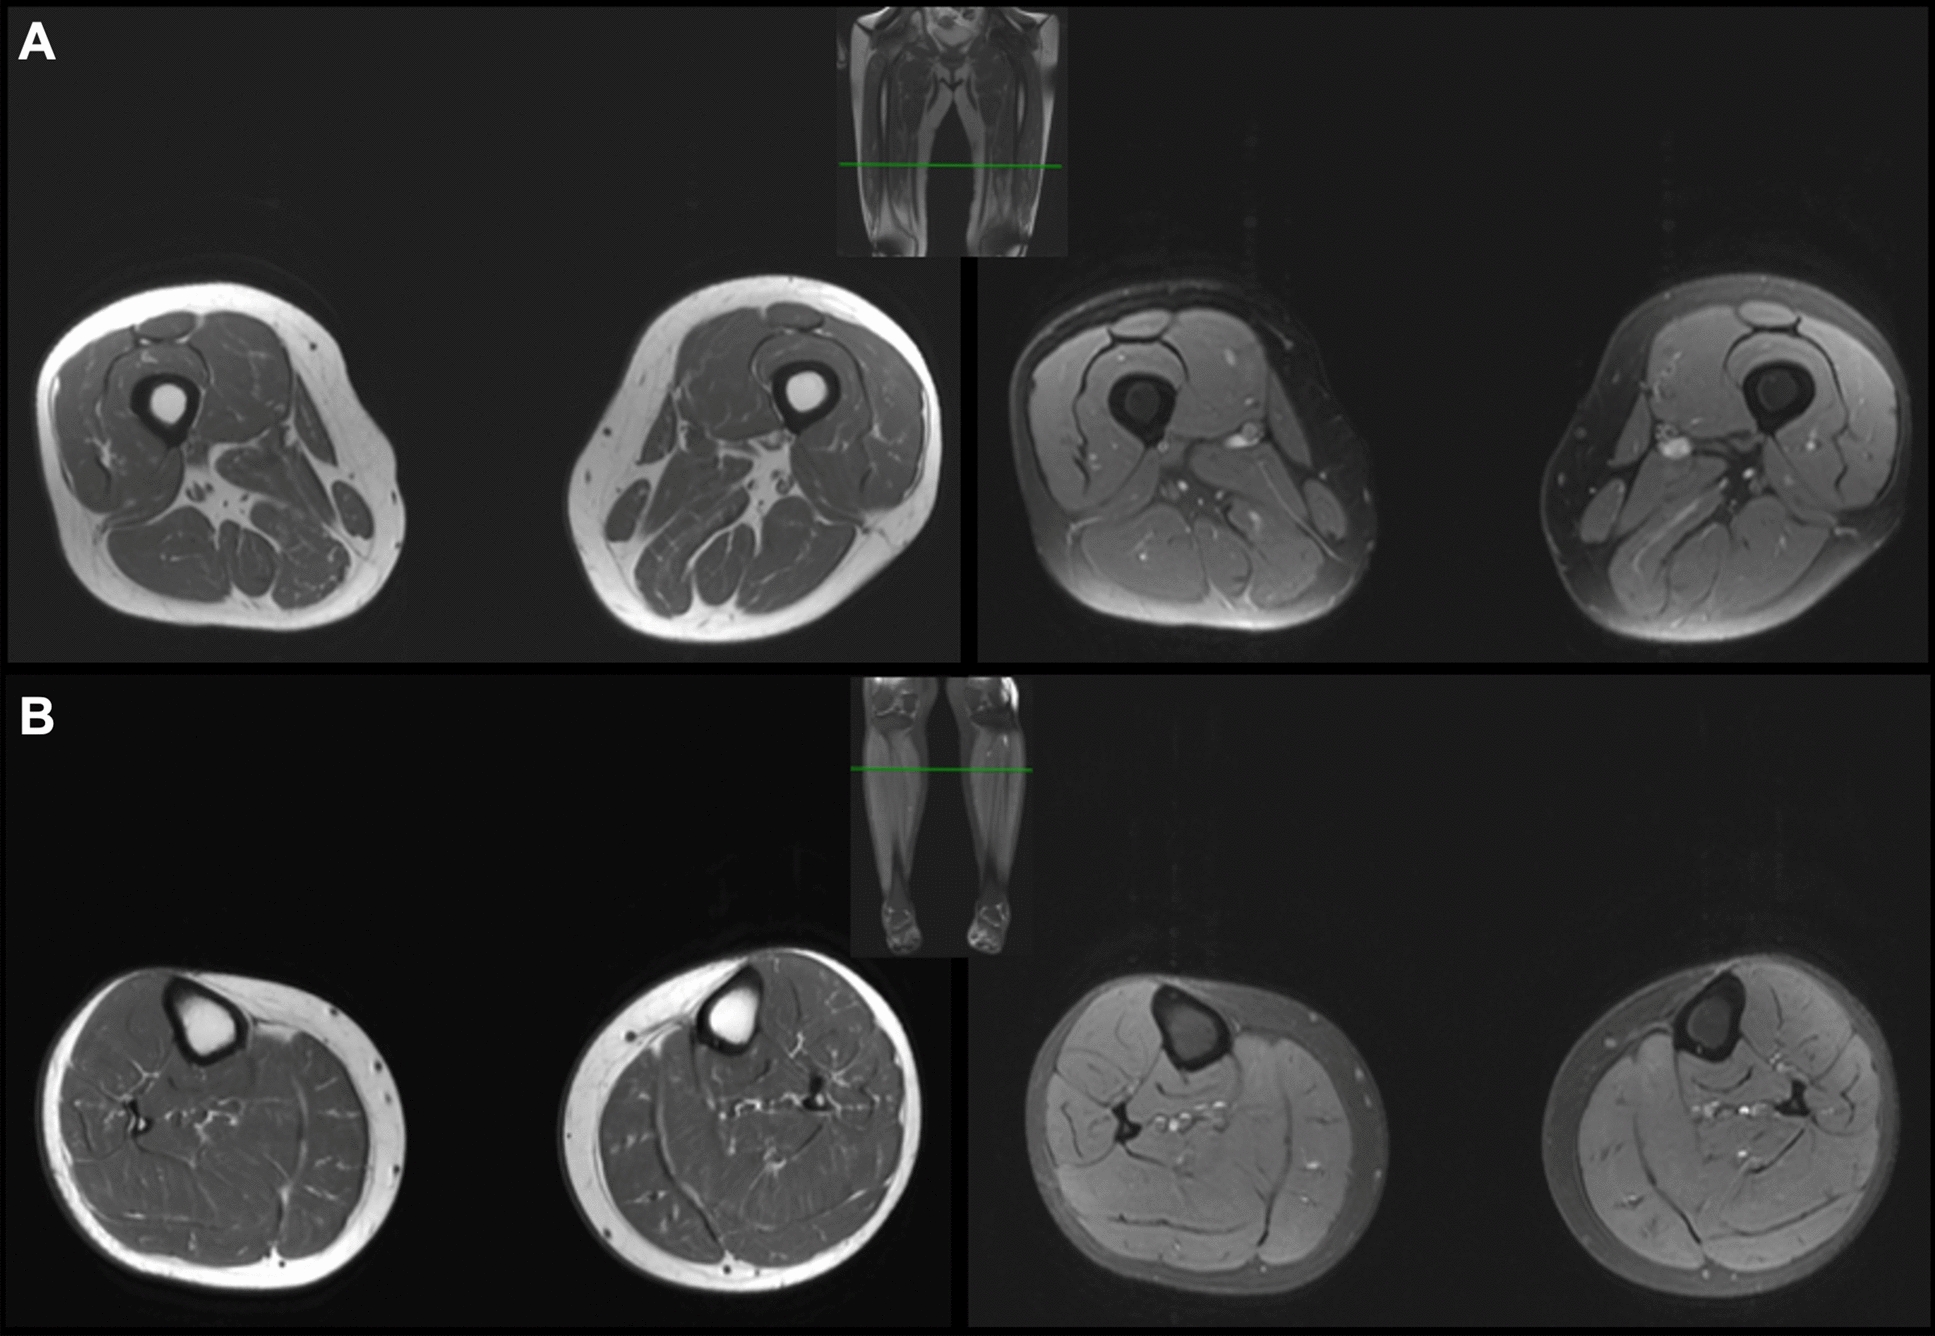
Patient 3's MRI images. A T1 non-contrast axial MRI of the bilateral lower thighs (left) and T2 STIR MRI (right) showing no muscle signal abnormalities. B T1 non-contrast axial MRI of the bilateral lower legs (left) and T2 STIR MRI (right) showing no muscle signal abnormalities
